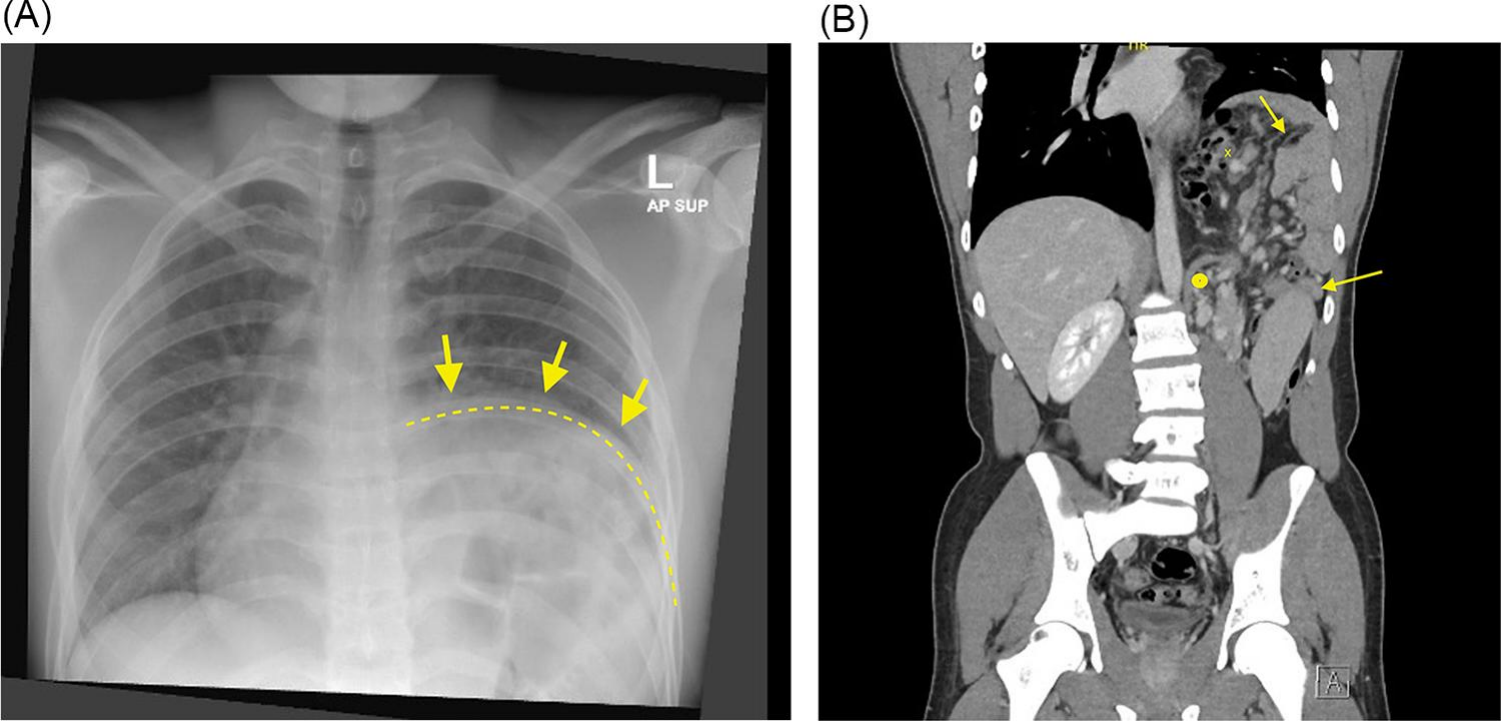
(A) Chest x-ray, AP view. Showing a large left diaphragmatic hernia with abdominal contents in the left hemothorax (arrows), and a mediastinal shift towards the right side. (B) Preoperative CT scan of the chest, abdomen, and pelvis with contrast, coronal view. Showing a left posterior defect on the left hemidiaphragm with herniation of small bowel loops, splenic flexure of the colon and left kidney with a hernia defect measuring about 5 × 7 centimeter (Arrow; splenic flexure of the colon. X; small bowels, *; left kidney).
